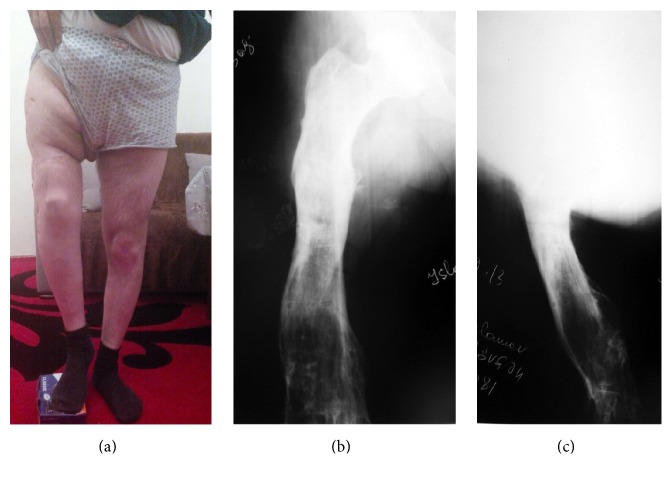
The patient was free of osteomyelitis and was able to walk with 4 cm shortness in the right lower extremity 7 years postoperatively. (a) Clinical appearance. (b, c) AP and lateral X-rays of the right femur seven years postoperatively.
